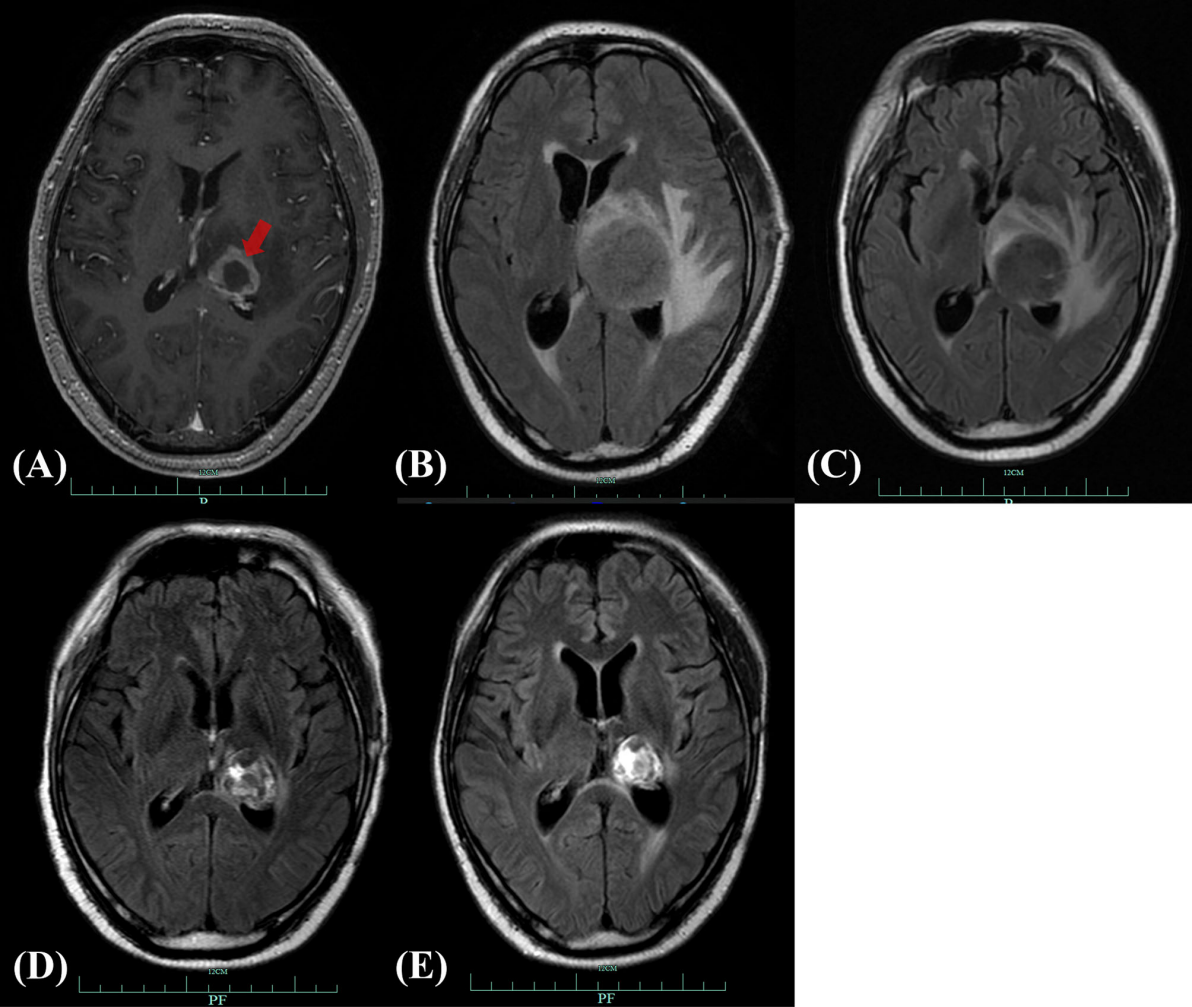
Brain MRI of CNS-PTLD. (A) Emergence of CNS-PTLD on day +225. MRI revealed a hypointensity lesion in the left thalamus with ring enhancement (red arrow) on contrast-enhanced T1-weighted imagining. (B) Day +255 (before treatment): the enlarged lesion surrounded by significant edema with the longest diameter of about 53mm (T2 Flair). (C) Day +280 (three weeks after the use of rituximab and MTX): reduction in the size of the lesion and edema with the longest diameter of 36.4mm (T2 Flair). (D) Day +363 (after whole-brain radiotherapy completed): further reduction in the size of the lesion with the longest diameter of 29mm (T2 Flair). (E) Day +477(three months after the start of zanubrutinib): the reduced lesion with the longest diameter of 24mm (T2 Flair). MRI, magnetic resonance imaging; CNS-PTLD, central nervous system post-transplant lymphoproliferative disorder.
